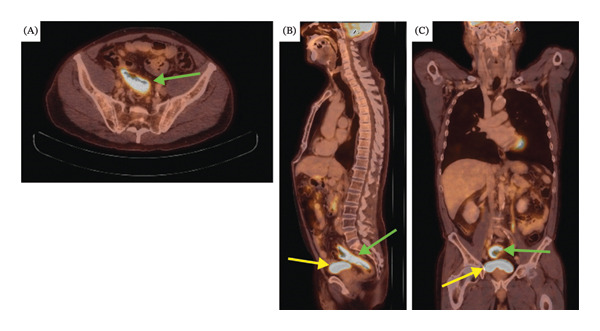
Repeat PET scan performed due to recurrence of symptoms. Images show increased FDG uptake in the colon as seen across the (A) coronal, (B) sagittal, and (C) axial planes. The green arrows point to the areas of colonic uptake. The yellow arrows indicate the normal activity of the bladder.
